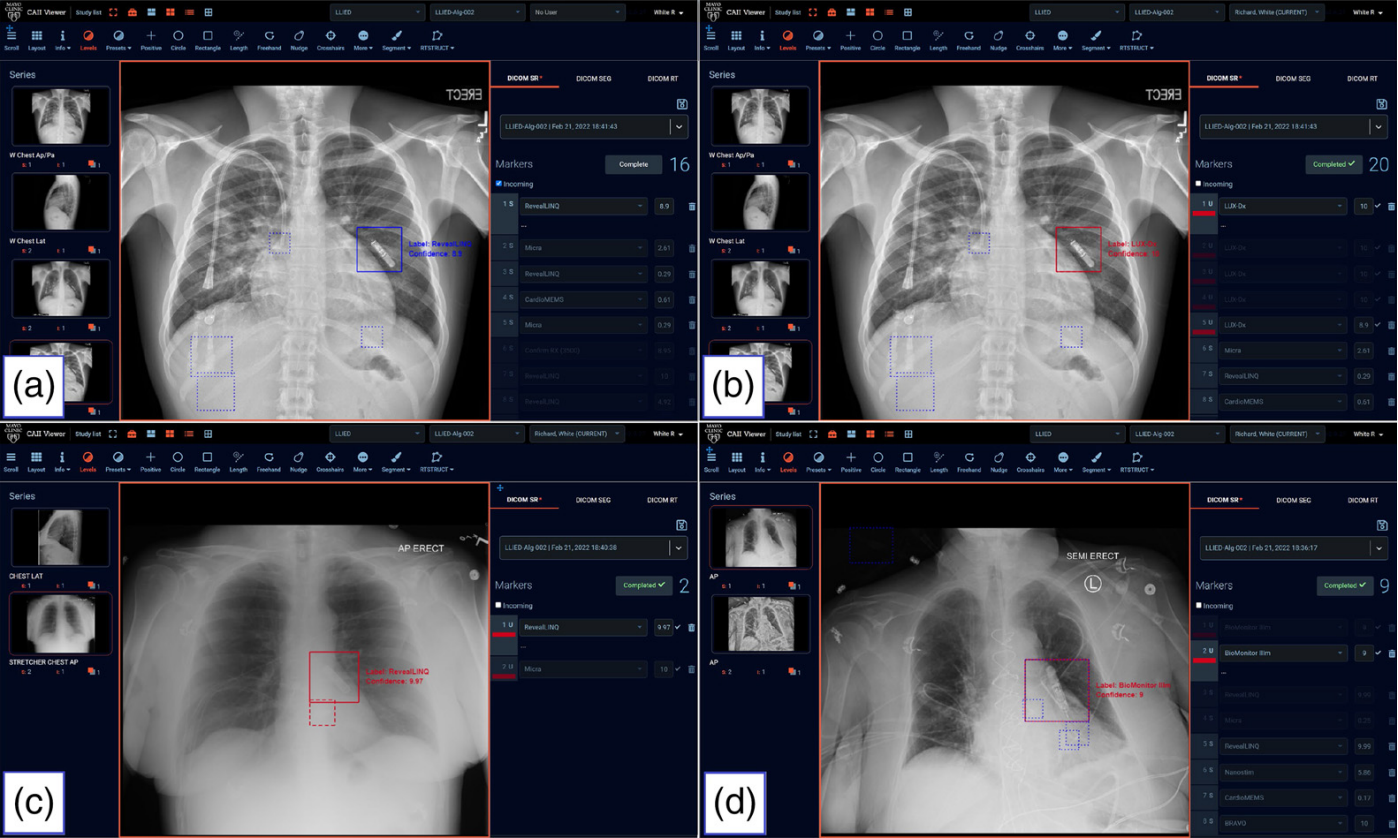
ZF GUI/viewer demonstrating inference results (location and probabilities) for end-user adjudication on three new LLIED types in methodology trial population. Previously, unclassified LLR types (i.e., A and B = LUX-Dx™; C = LINQ™ II; D = BioMonitor III) were properly detected as simple conditional LLRs by the original LLIED model, although misidentified as the most common LLR (i.e., Reveal LINQ™) of equal MRI safety (i.e., simple conditional). A previously classified assessment-requiring conditional LLP (i.e., C = Micra™) was both correctly detected and identified at a high probability level, with an appropriate inference GBB label automatically assigned. The user-friendly inference-adjudication capabilities of the ZF GUI/viewer allowed manual label reassignment of inference GBB labels from a drop-down list (e.g., Reveal LINQ™ in A relabeled as LUX-Dx™ in B), or confirmation of correct automatic assignment (e.g., Micra™ in C). In addition, an “other” option for labeling of false-negative or unanticipated future LLIEDs by the end-user is also included. All end-user adjudications of inference results are recorded in the ZF GUI/viewer backend database in support of model continuous learning and modernization.
